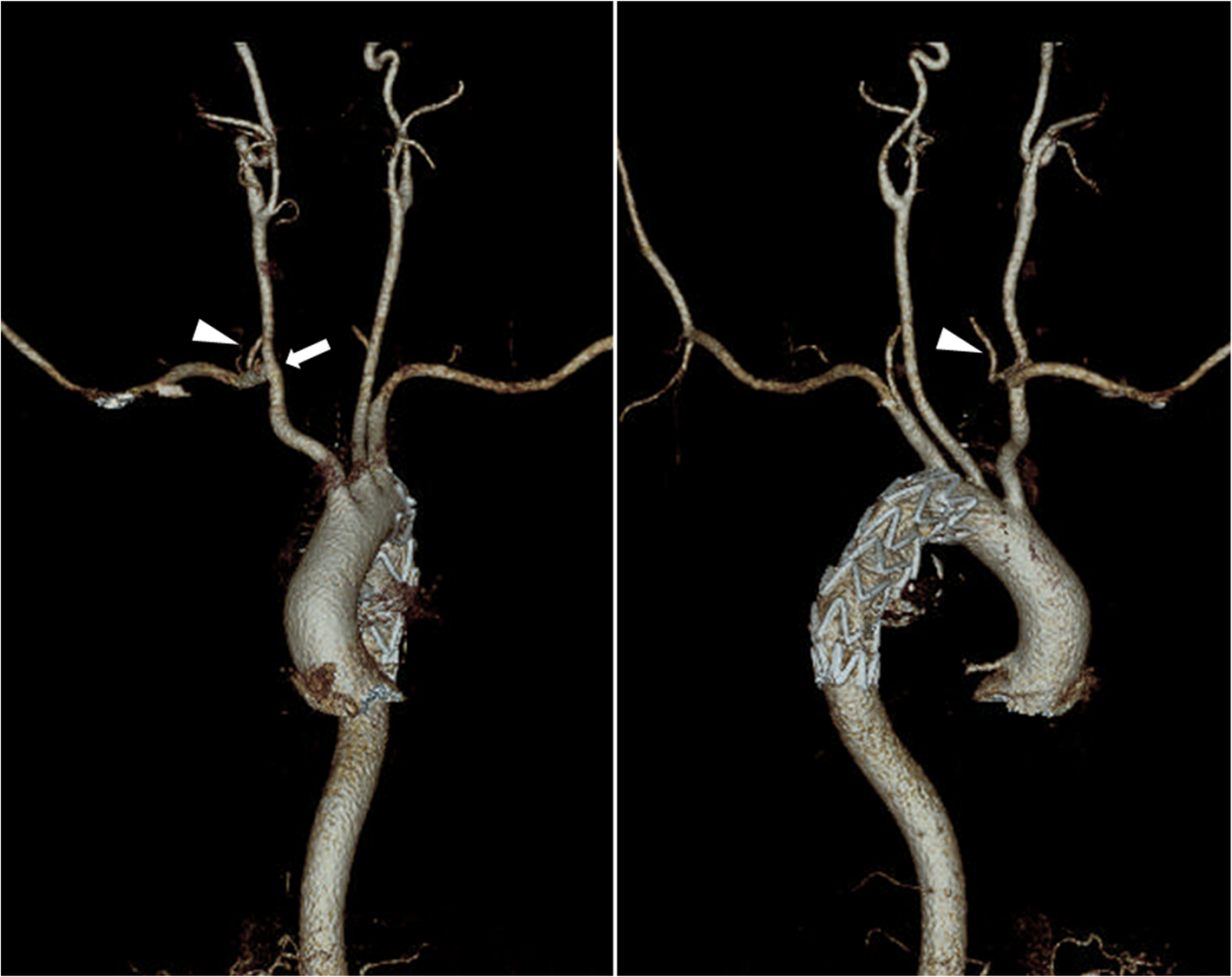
Computed tomographic angiography showing the result of hybrid treatment with transposition of right subclavian artery in the right common carotid artery (arrow) with intact right vertebral artery vertebral (arrow head) and thoracic endovascular aneurysm repair with complete occlusion of ARSA
